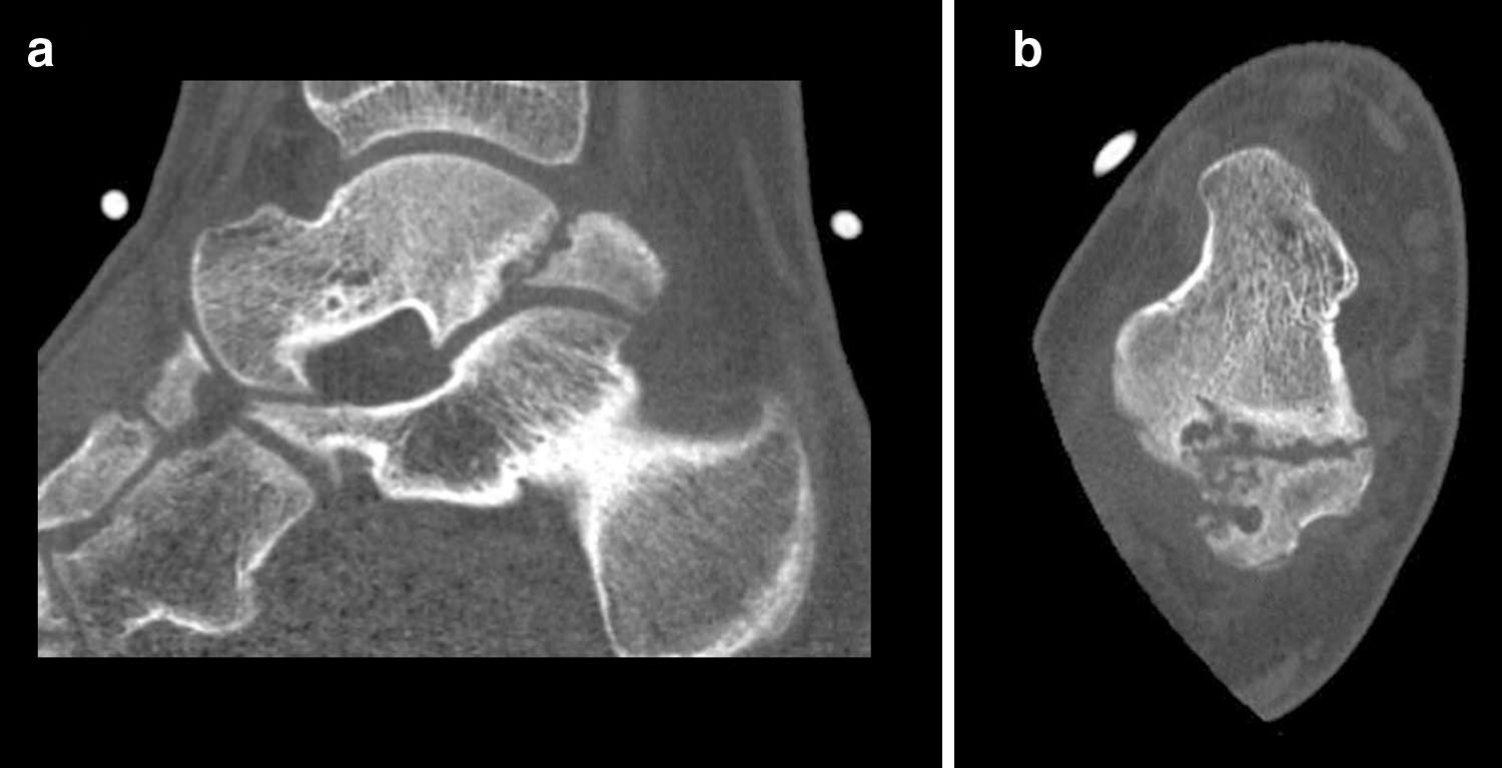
CT scan case 2. a Sagittal view indicating talus bipartitus with degenerative changes. b Axial view of the right ankle
